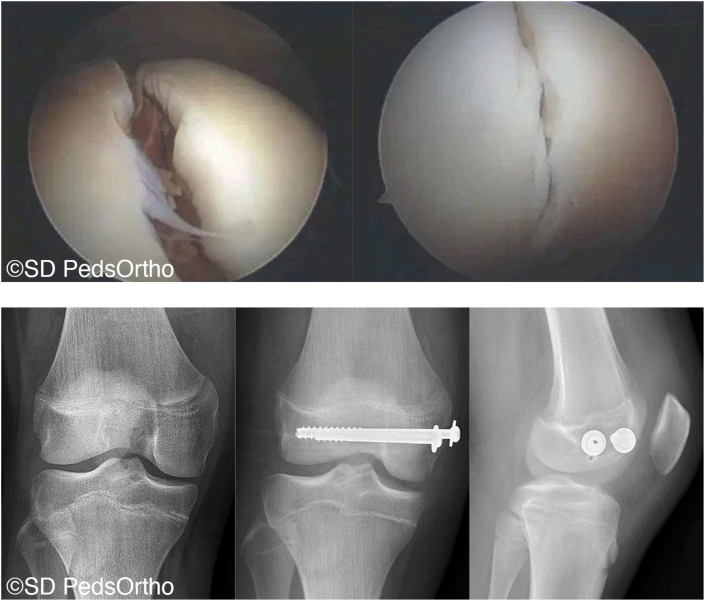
TOP: Preoperative (left) and postoperative arthroscopic images (right). BOTTOM: Preoperative (left) and postoperative radiographs.
